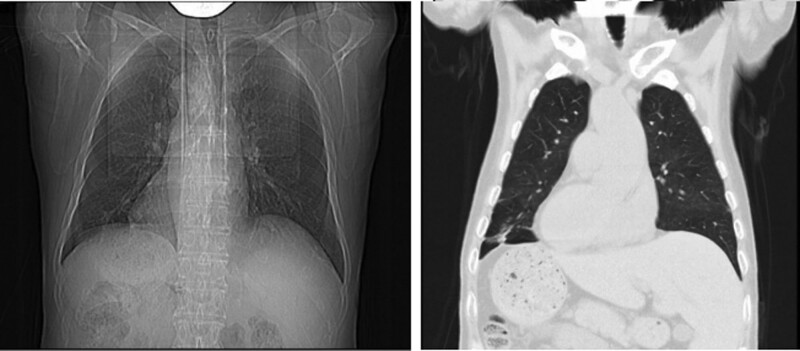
The patient’s chest X-ray and CT showed a mirror-image transposition of both the abdominal and the thoracic organs.
